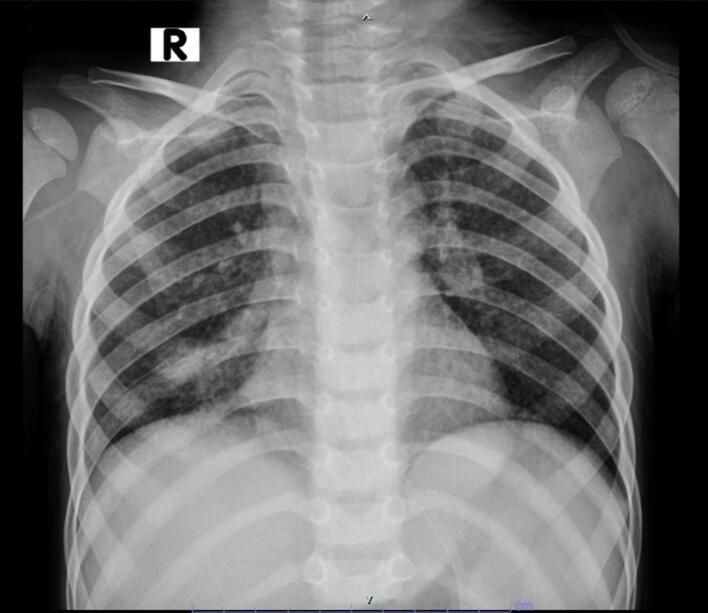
Chest X-ray revealed a right parahilar infiltrates.
